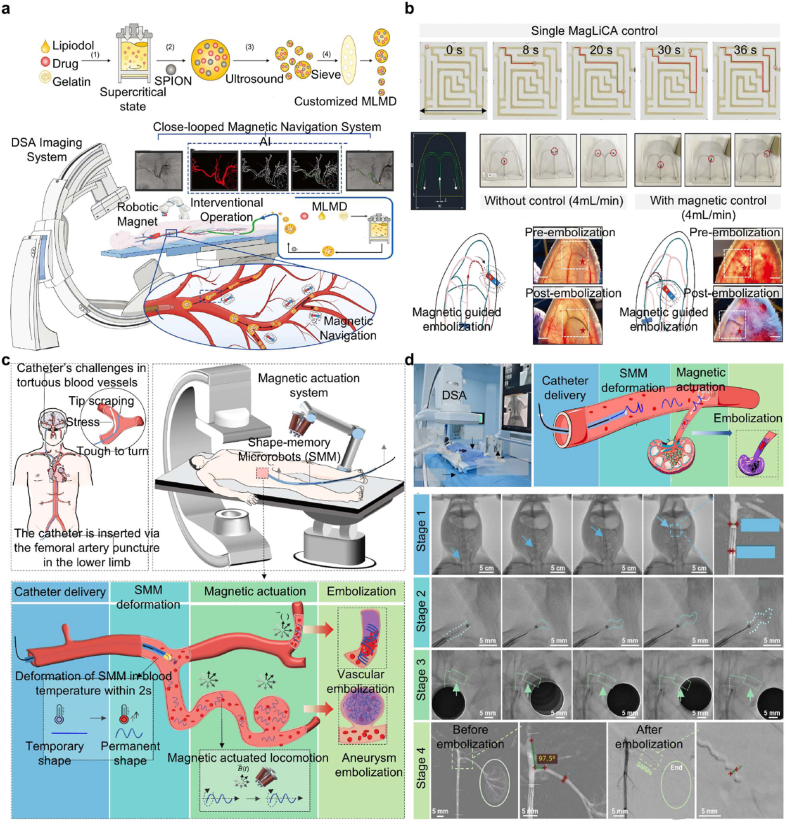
Embolic hydrogel robots achieve intelligent and precise tumor embolization. (a) MLMDs efficiently perform navigation tasks, accurately targeting lesions under the control of an external magnetic field to achieve precise embolization. Reproduced with permission from Ref. [184]. Copyright 2025, Wiley-VCH. (b) MagLiCA microrobots, highly resolvable under X-ray imaging, are expected to enable precise drug delivery and treatment through real-time. Reproduced with permission from Ref. [185]. Copyright 2024, Wiley-VCH. (c) SMMs can overcome narrow and tortuous vascular obstacles under a rotating magnetic field, moving flexibly through blood vessels to achieve multi-level vascular embolization. Reproduced with permission from Ref. [186]. Copyright 2024, American Association for the Advancement of Science. (d) SMMs enable rapid and efficient renal embolization. Reproduced with permission from Ref*.* [186]*.* Copyright 2024, American Association for the Advancement of Science.
